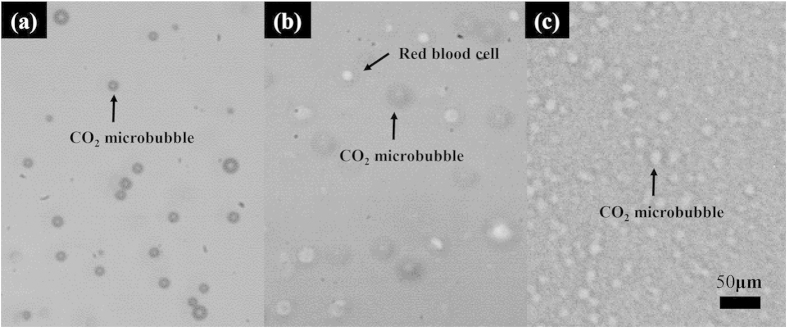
Optical image of (a) CO_2_ micorbubbles and (b) CO_2_ microbubbles with 2% HCT blood. (c) Typical X-ray image of CO_2_ microbubbles with 40% HCT blood.
